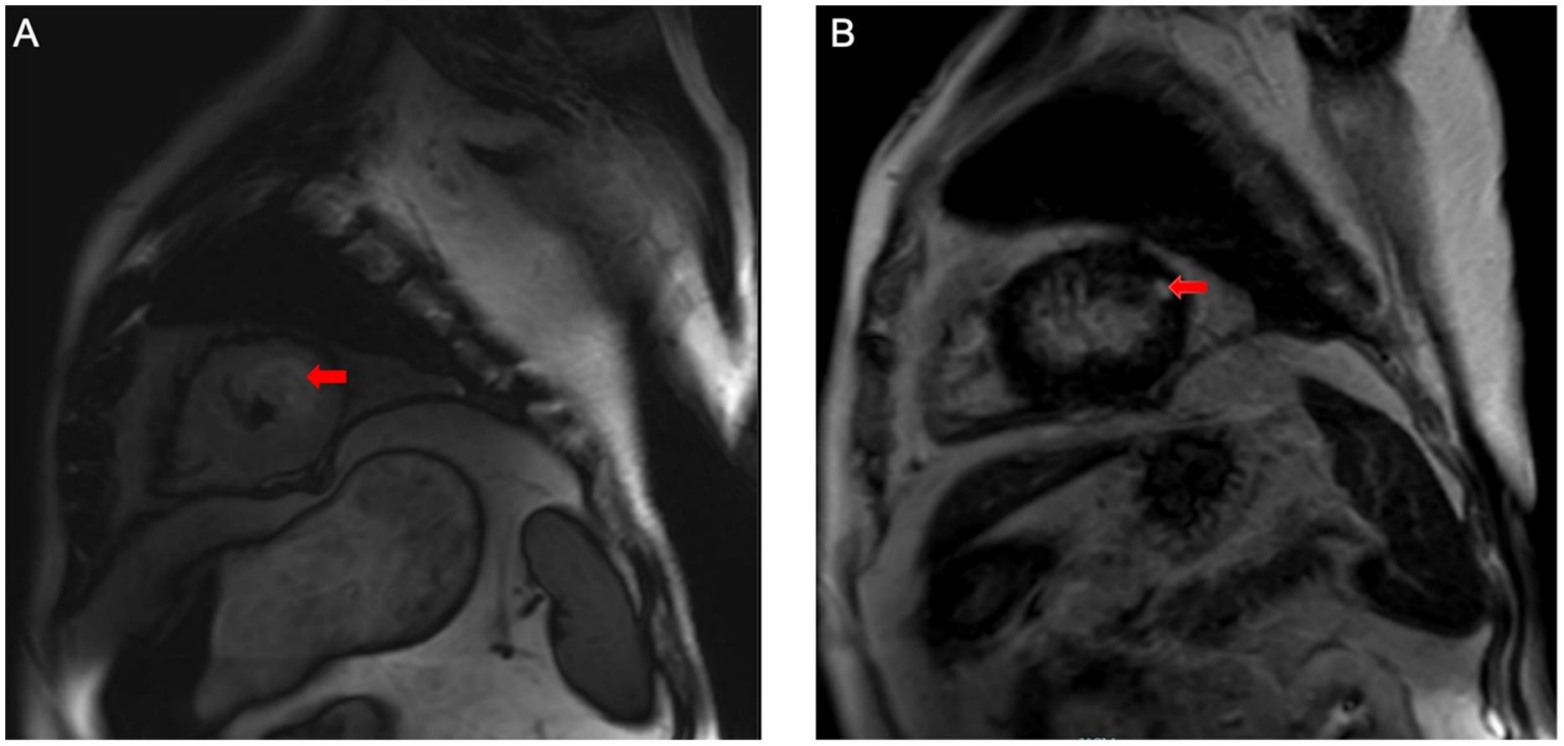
The results of the patient's cardiac MRI. (A) On the T2-STIR sequence, there is mild edema in the apical region of the heart (red arrow). (B) On the late gadolinium enhancement sequence, mild fibrosis can be observed in the apical region of the heart (red arrow).
